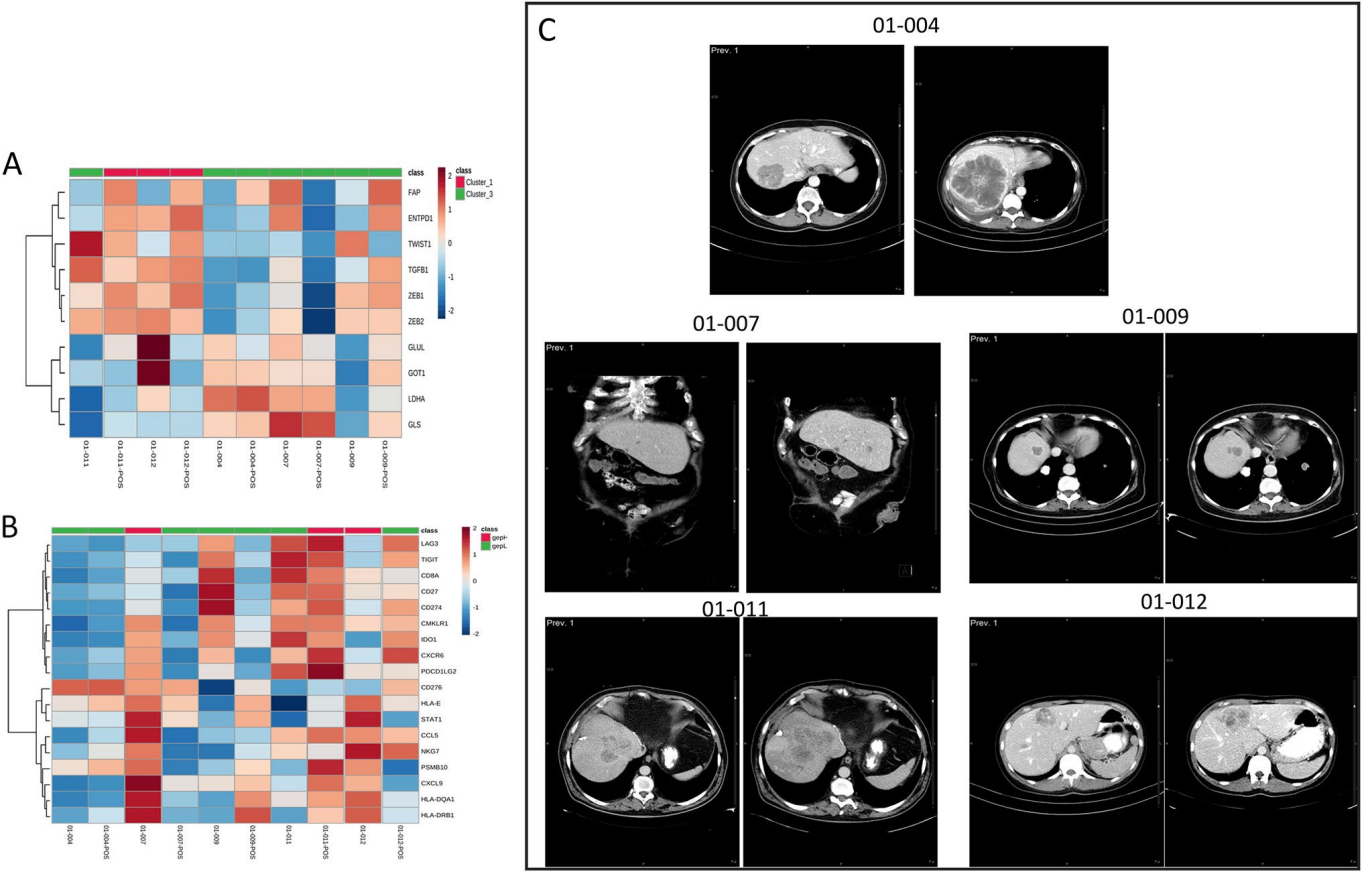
Liver biopsies before and after therapy (2-month evaluation) analyzed in both signatures. 3A. Changes in gene expression with IMMETCOLS signature. 3B. Changes in gene expression with GEP signature. 3C. Radiological CT basally and at 2 month therapy
